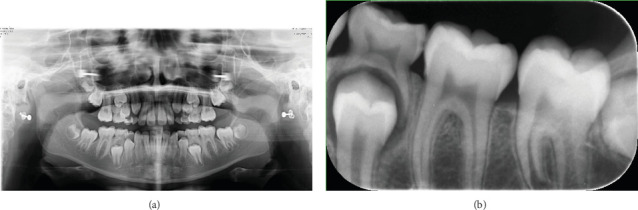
(a) The radiographic findings in the panorama image were insignificant. (b) The radiographic findings in periapical image were insignificant.
